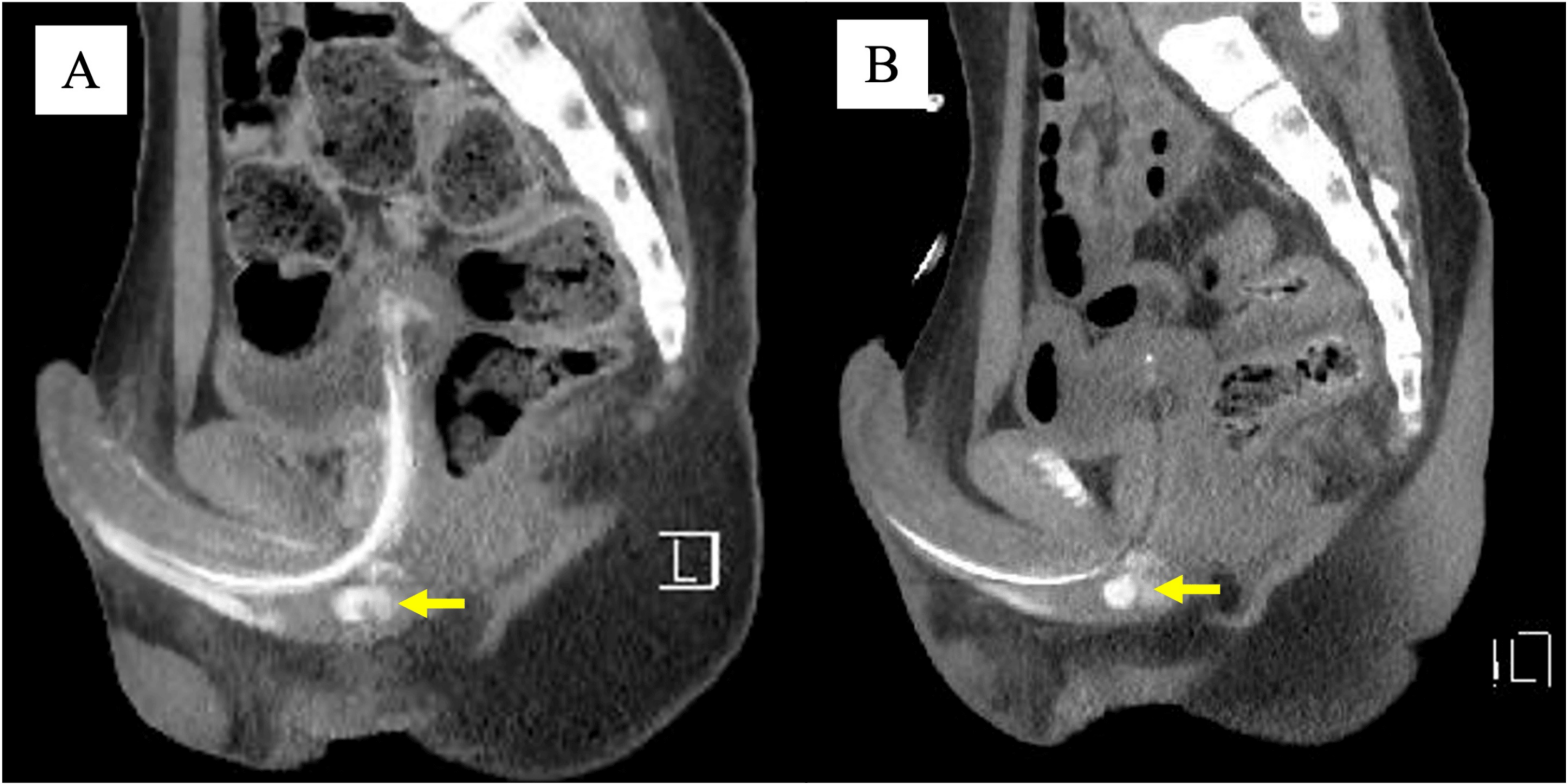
Contrast-enhanced CT findings of traumatic urethral pseudoaneurysm. (A) Contrast-enhanced CT showing contrast extravasation from the distal bulbar artery (the yellow arrow indicates the urethral pseudoaneurysm). (B) Contrast-enhanced CT obtained two days after transcatheter arterial embolization demonstrating recanalization (the yellow arrow indicates the urethral pseudoaneurysm).
